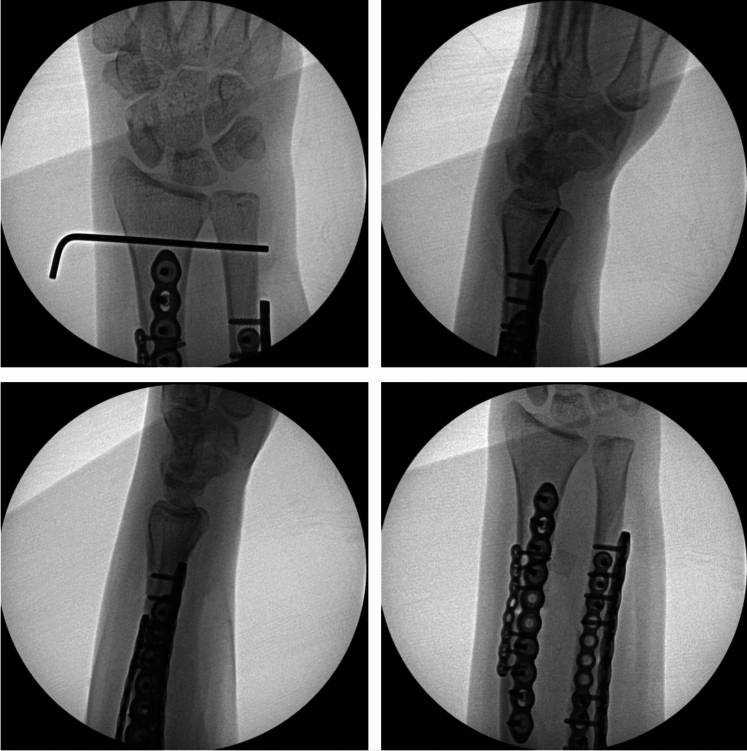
Intraoperative radiographs demonstrating reduced DRUJ and neutral ulnar variance after radial osteotomy and fixation of both the radius and ulna
